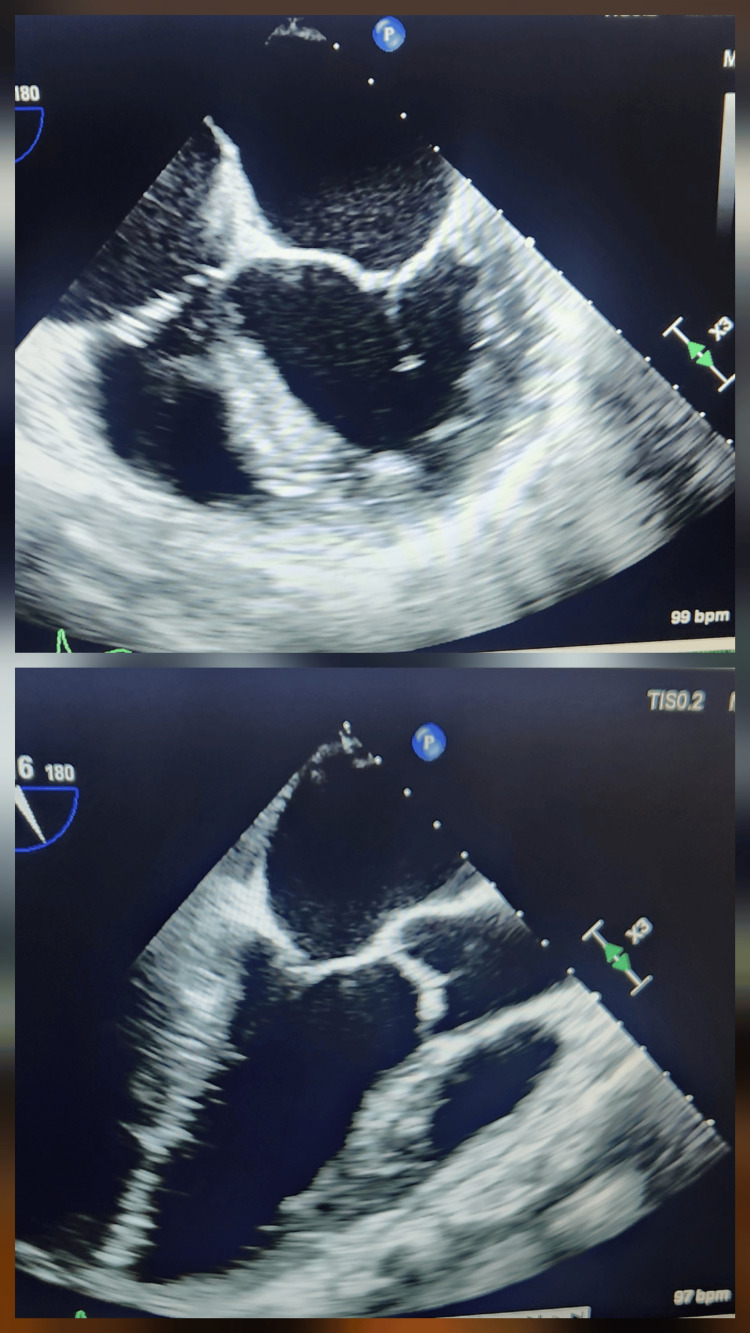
Transesophageal echocardiography demonstrating normal mitral and tricuspid valves, with no evidence of valvular vegetations or intracardiac thrombus, effectively excluding infective endocarditis in the setting of persistent methicillin-resistant Staphylococcus aureus bacteremia.
